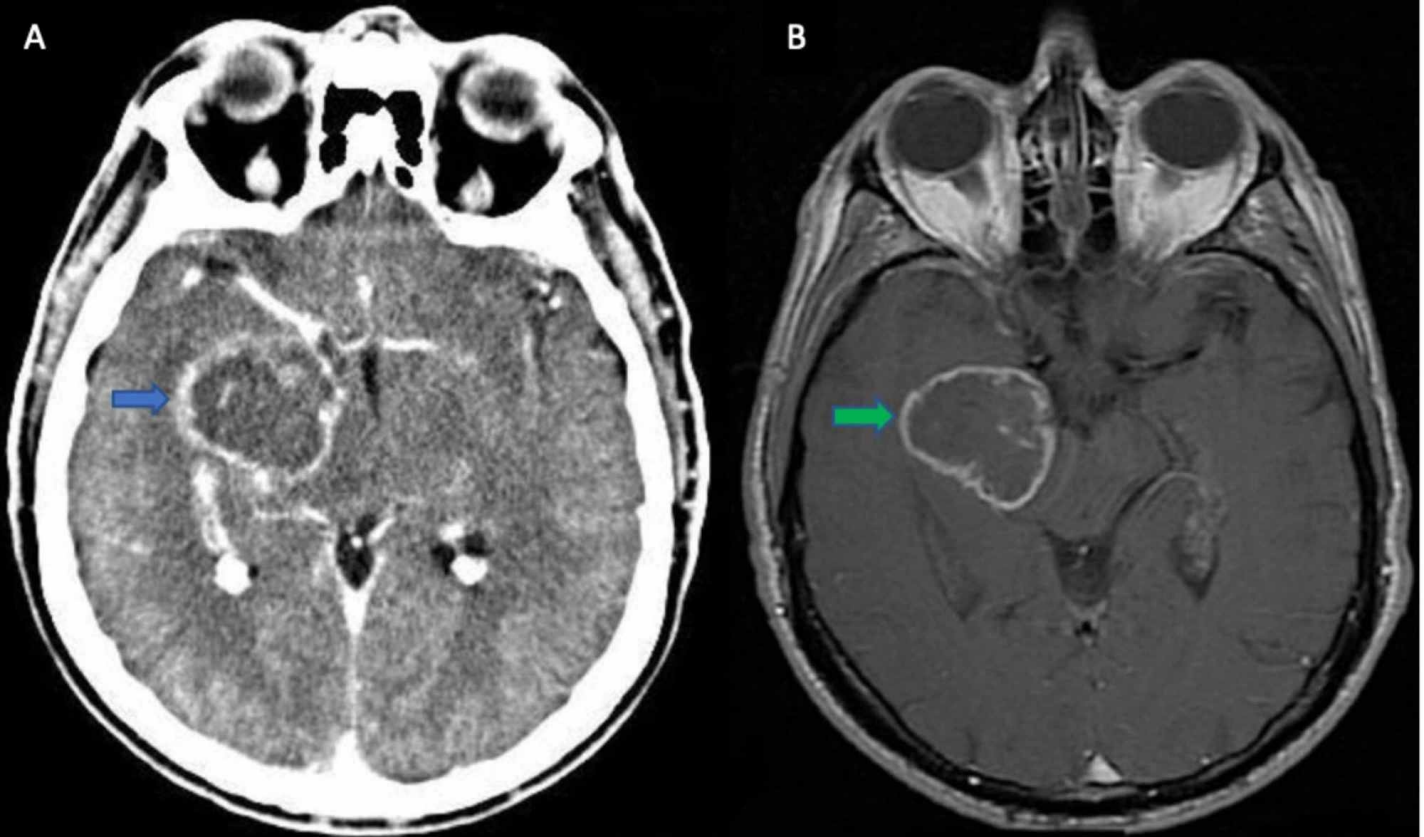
(A) CT brain axial with contrast showing a right temporal lobe tumor with an irregular ring enhancement (blue arrow). (B) MRI brain -T1 axial gadolinium (Gd) showing probably a high-grade glioma (green arrow)
